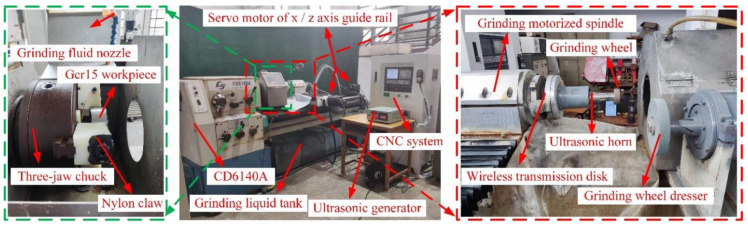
The longitudinal-torsional ultrasonic vibration grinding experiment.
